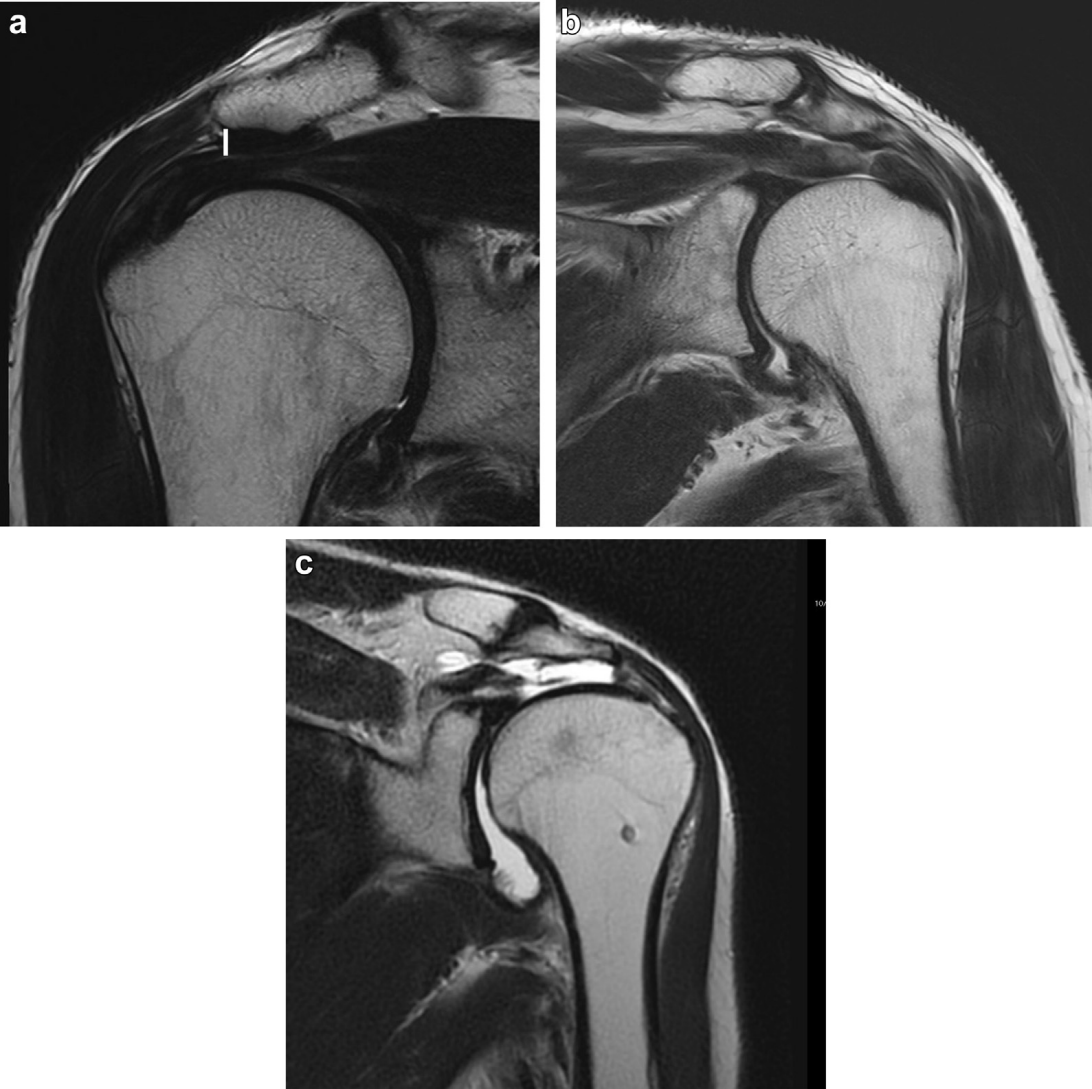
(a) The oblique coronal section of an MRI image showing measurement of the CAL thickness at the acromial undersurface. The CAL thickness was measured at the lateral margin of the acromion at the insertion of the CAL. A vertical line was drawn at the thickest point of the CAL insertion. (b) The oblique coronal section of an MRI image showing a thick subacromial CAL with a deep bursal-side partial-thickness rotator cuff tear (CAL thickness: 3.9 mm). (c) The oblique coronal section of an MRI image showing a thin subacromial CAL with a large rotator cuff tear and a thin subacromial CAL (CAL thickness: 0 mm). *MRI*, magnetic resonance imaging; *CAL*, coracoacromial ligament.
